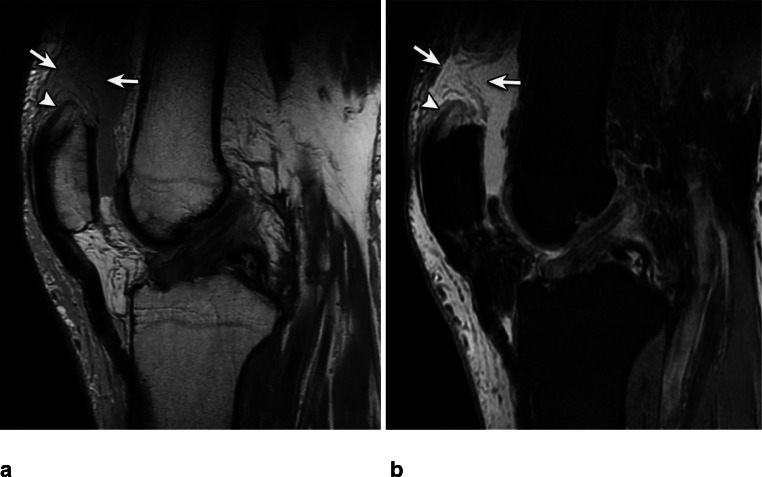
Eighty-three-year-old male with quadriceps femoris tear. Intermediate-weighted MR images without (a) and with (b) fat saturation show a complete quadriceps tendon rupture proximal to the patella (arrows) with a tendon stump (arrowhead). The patient was treated surgically with transpatellar bone tunnel tendon fixation
